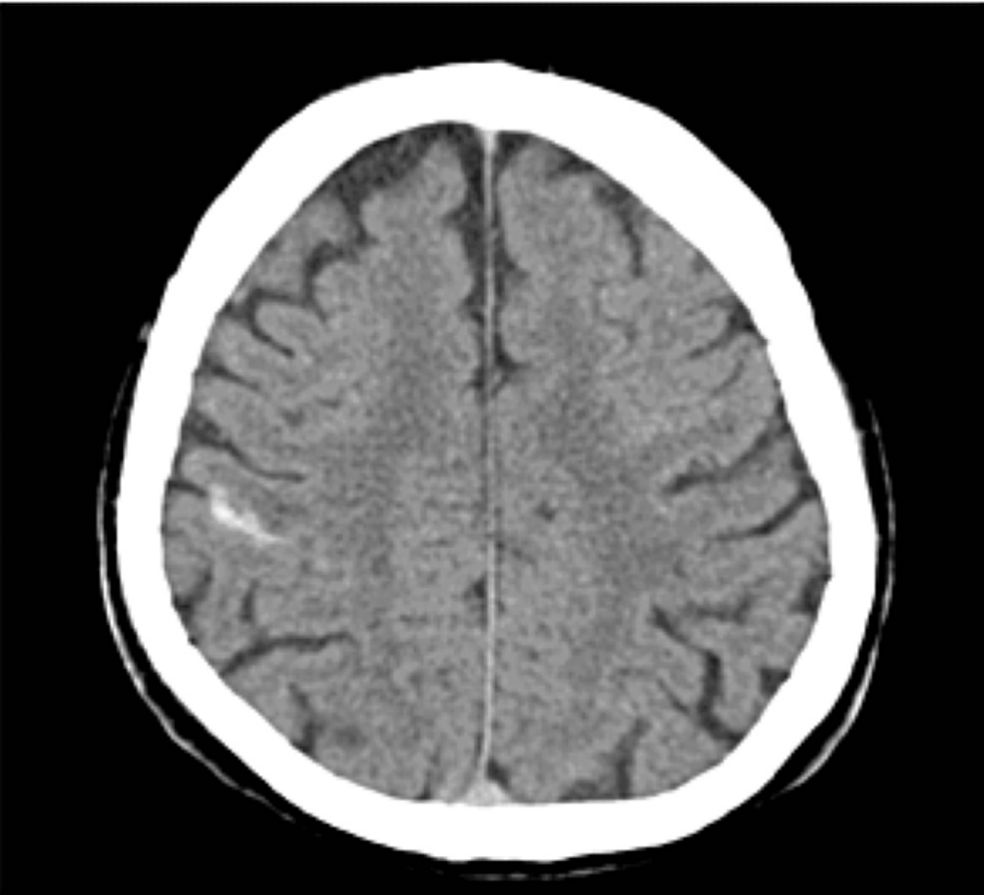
Brain CT scan Head CT scan showing a focal hemorrhage filling the right postcentral sulcus associated with hypodensity in the postcentral gyrus corresponding to ischemia or edema.
